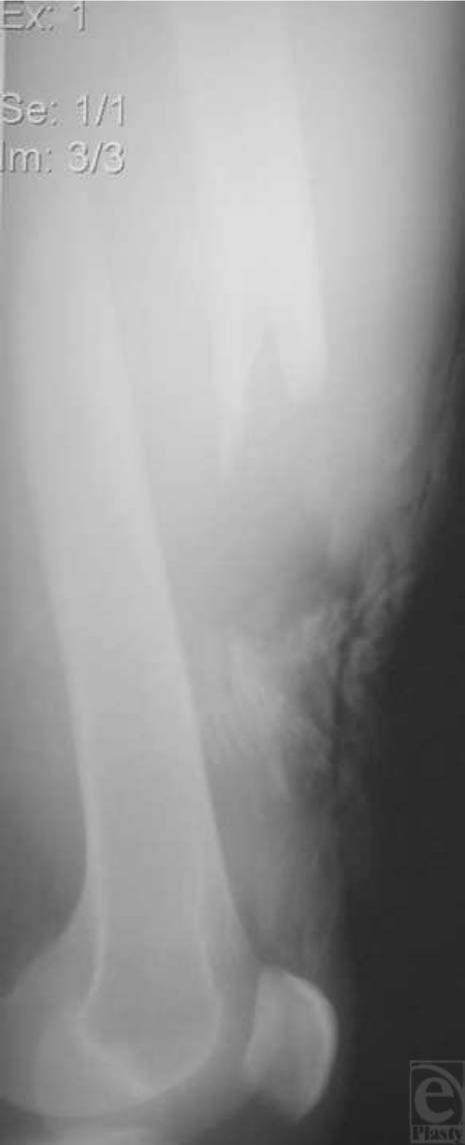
Lateral radiograph of the right femur shows a type IIIB open fracture. Note the soft tissue burn injuries adjacent to the fracture.
